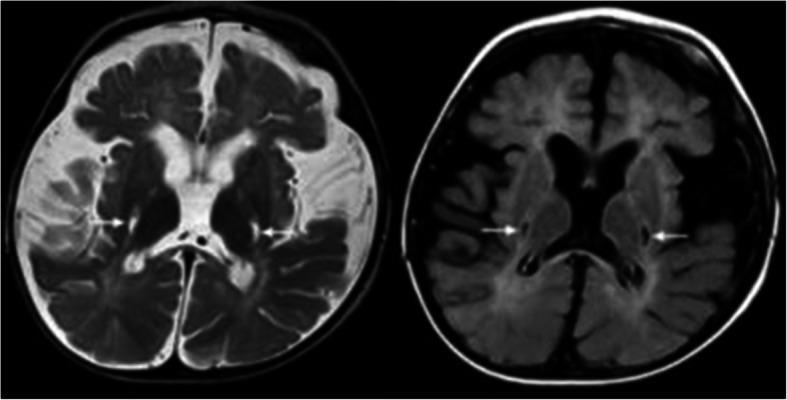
Pattern A1 (early-infantile form) at an advanced stage. Axial images (T2w left, Flair right) show cystic degeneration of the pyramidal tract (white arrows), severe atrophy with enlarged insulae and diffusely affected white matter. Patient 12 (onset 4 months, age at MRI 8 months).
